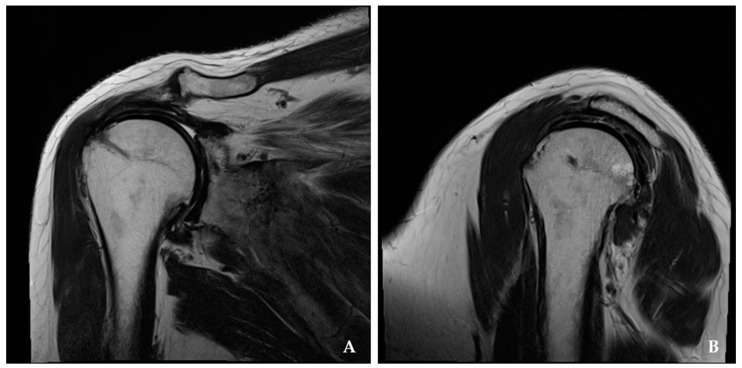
Superior capsular reconstruction with the acellular dermal allograft is shown at the postoperative 1 year. (A) magnetic resonance imaging of T1 coronal section. (B) magnetic resonance imaging of T1.
